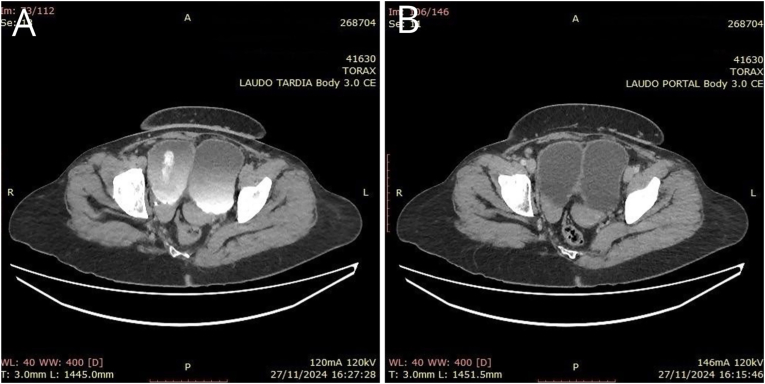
Computed tomography, axial plane: A - Uterus didelphys. B - Duplicated bladder.
